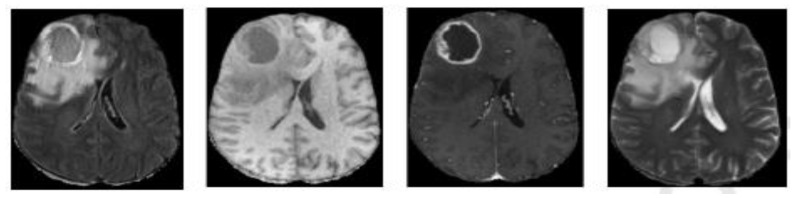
Different modalities of an MRI image from left to right: Flair, T1, T1ce, and T2.
